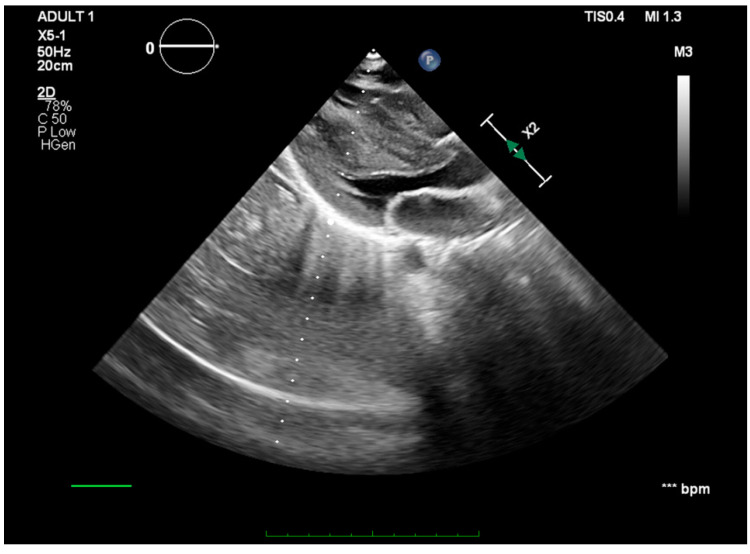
Parasternal long-axis view—the intracardiac tumor protrudes through the aortic valve during systole.
